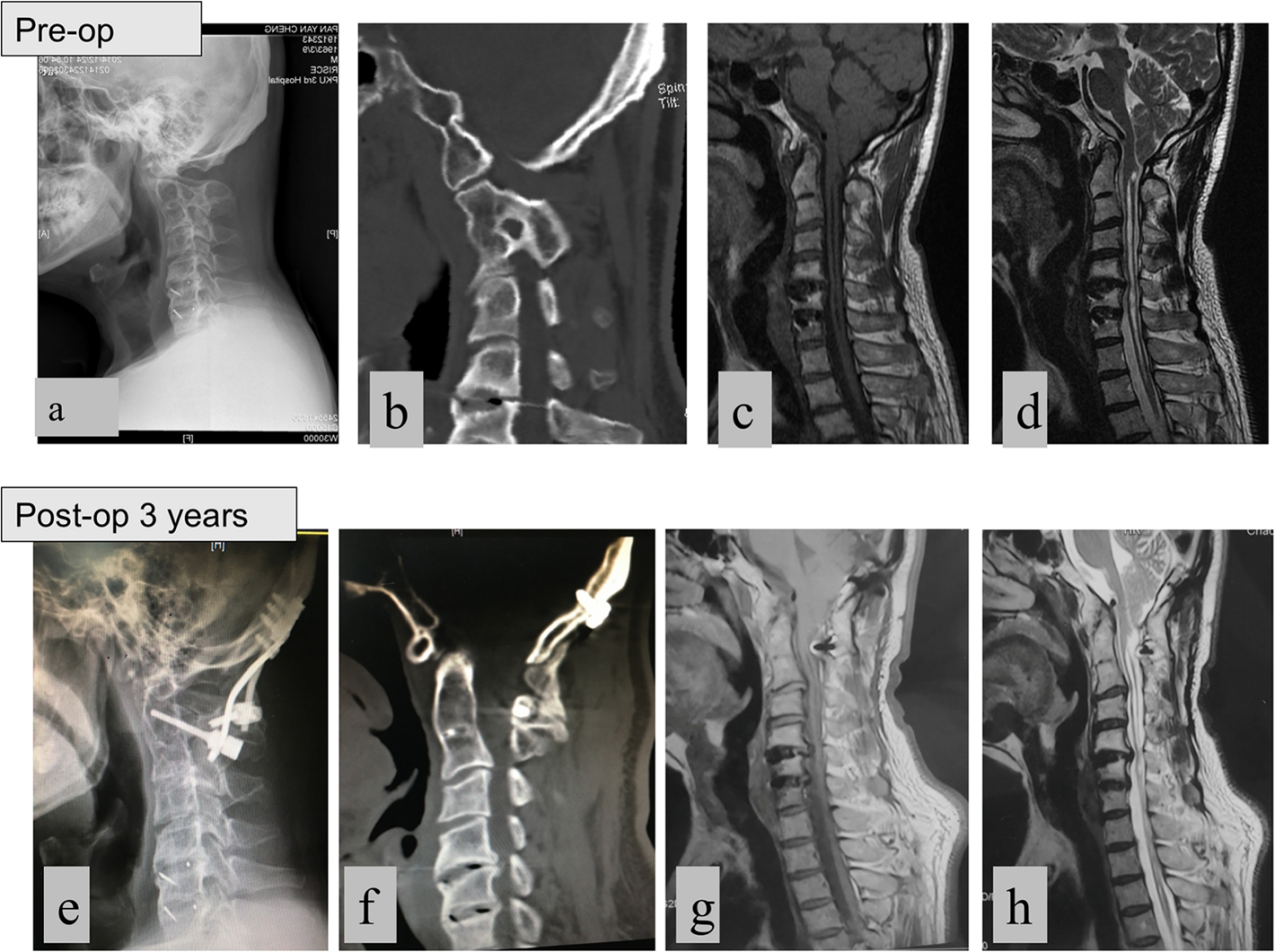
A 51-y/o male patient with severe myelopathy. a lateral radiograph showed sandwich AAD, and ADI = 5.5 mm; b CT indicated C1–2 facet remodeling (sloped from horizontally to vertically); c and d MRI showed Chiari deformity and syringomyelia from C2 to T2; e C2 laminar screw and pedicle screw fixation on 3-year follow-up radiograph (lateral); f 3-year follow-up CT showed the bony fusion between the occiput and the axis; g and h: 3-year follow-up MRI showed the partial relief of Chiari deformity and syringomyelia
